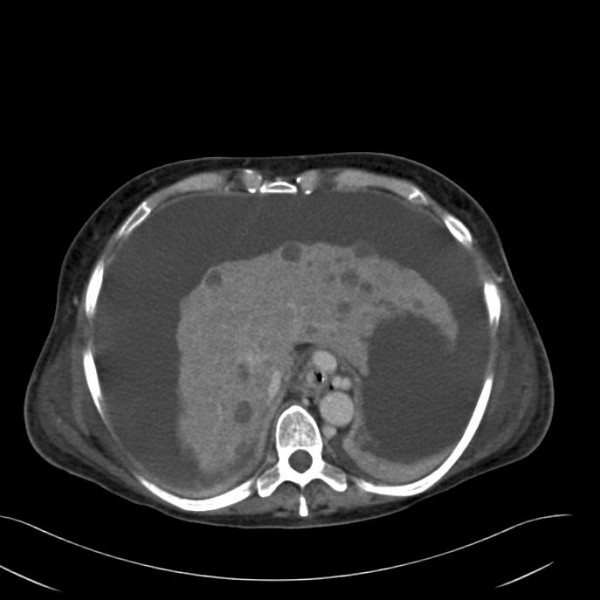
CT scan with multiple new hypodense lesions.
